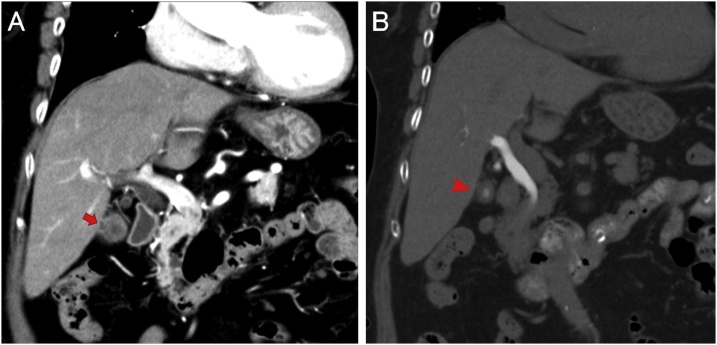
Radiological findings. (A) A cystic lesion (red arrow) was noted in the wall of the gallbladder via enhanced abdominal computed tomography (CT). (B) The cyst was not enhanced by drip infusion cholecystocholangiography-CT (red arrowhead).
